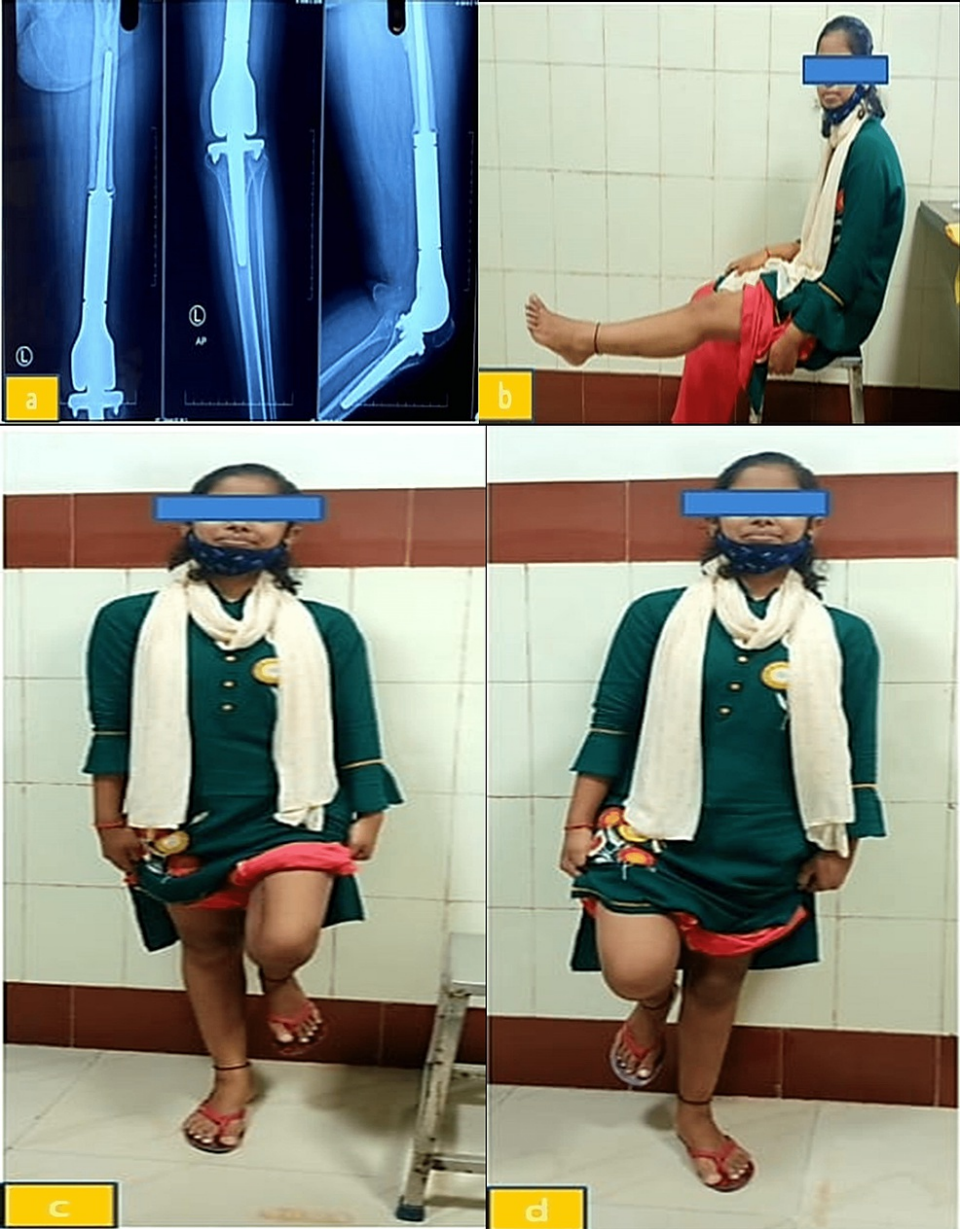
Outcome of limb salvage surgery for osteosarcoma of the distal femur with hinge knee mega prosthesis. (a) X-ray of the left limb following limb salvage surgery for osteosarcoma of the distal femur with hinge knee mega prosthesis at two-year follow-op. (b) Full extension of knee in the left prosthetic limb. (c) Standing on the normal right leg with 90-degree flexion in the left prosthetic limb. (d) Standing on a prosthetic left limb with 90-degree flexion in the normal right leg.
